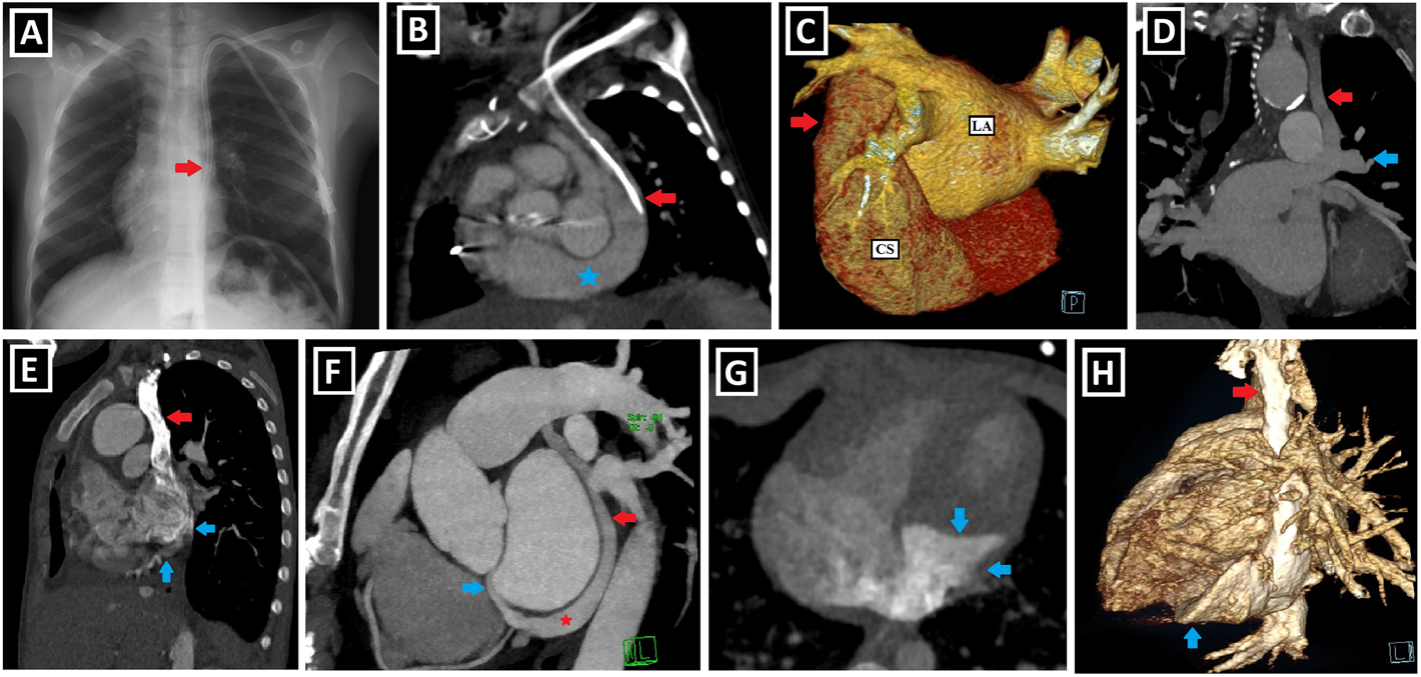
Clinical importance and different drainage sites of PLSVC and possible anatomical changes. a, b Posterior-anterior (PA) chest X-ray (a), and sagittal-oblique reformatted CT image (b) of different patients depict the course of central venous catheters inserted into the PLSVC (red arrows) draining into the right atrium via the coronary sinus (blue star). c CT imaging performed to evaluate the cardiac anatomy and possible variations of pulmonary veins draining to the left atrium (LA) before the radiofrequency catheter ablation in a patient with atrial fibrillation. The three-dimensional volume rendering technique (3D VRT) image shows that PLSVC (red arrow), which is detected incidentally, drains to the coronary sinus (CS). d Coronal-oblique maximum intensity projection CT image indicates PLSVC (red arrow), which indirectly drains into the left atrium via the left upper pulmonary vein (blue arrow). e Sagittal-oblique reformatted CT image shows PLSVC (red arrow) draining into the left atrium via the unroofed coronary sinus (blue arrows). Since ASD (not shown) is also present, the findings are compatible with Raghib syndrome. f Sagittal-oblique maximum intensity projection CT image demonstrates that the coronary sinus is connected to the left atrium with an aberrant vein as a collateral drainage pathway in a patient with coronary sinus ostial atresia (not shown). Thus, PLSVC (red arrow) drains into the left atrium through the coronary sinus (red star) and interatrial aberrant vein (blue arrow). g, h Axial CT (f) and 3D VRT images with different views (g, h) indicate the coronary sinus aneurysm (blue arrows) in a patient with PLSVC (red arrow) draining into the coronary sinus
